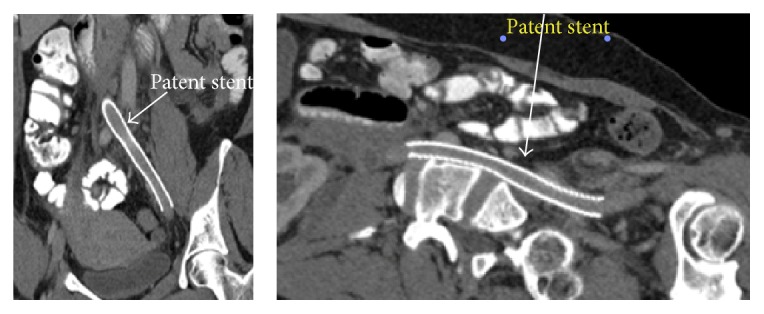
Follow-up CT scan showed patent iliac vein stent.
